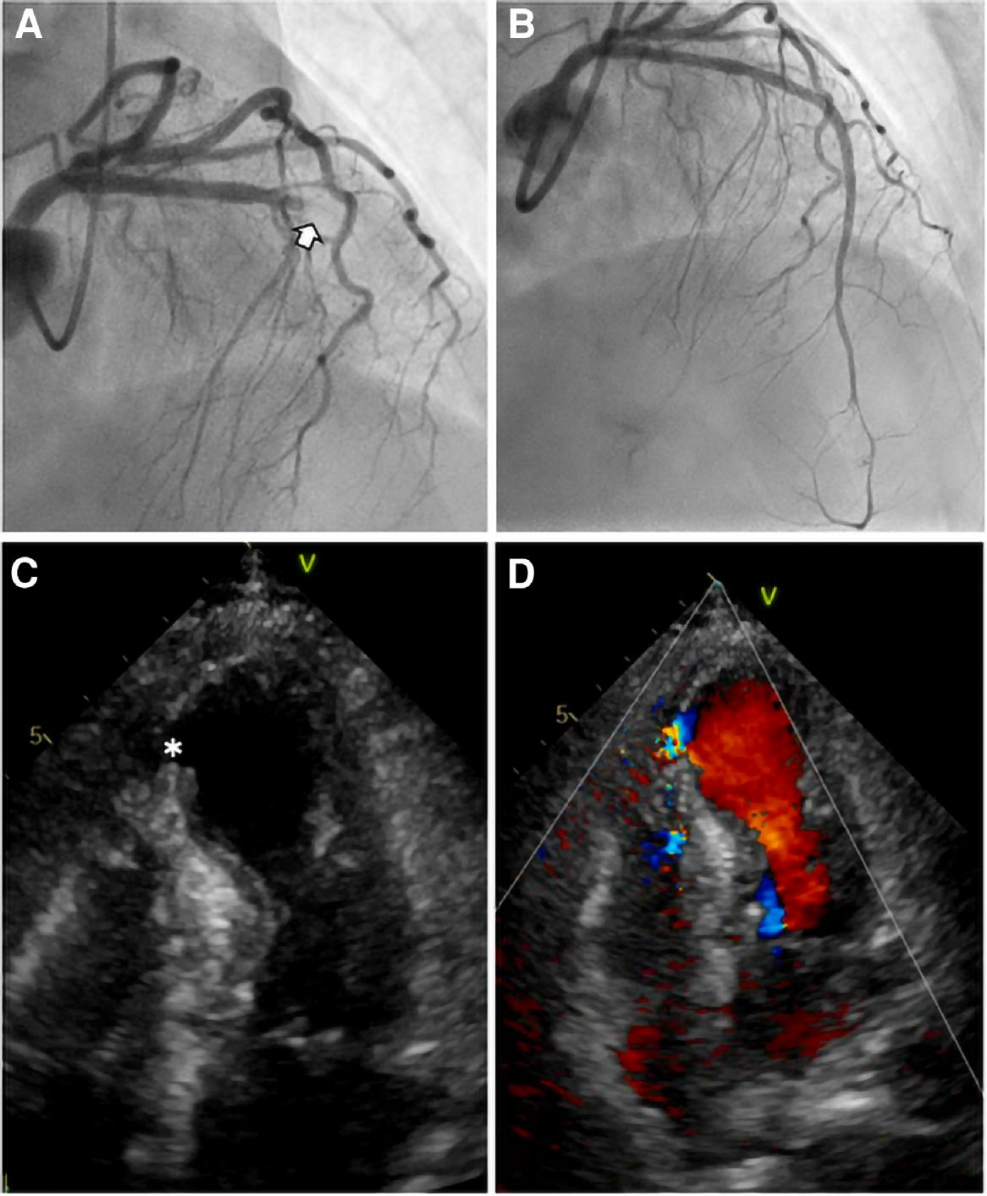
Imaging of acute left anterior descending artery infarction and ventricular septal rupture. (*A*) Coronary angiography showing total left anterior descending artery occlusion (arrow). (*B*) Successful revascularization following drug-eluting stent implantation. (*C*) Transthoracic echocardiography revealing an apical ventricular septal rupture (asterisk). (*D*) Colour Doppler image showing a left-to-right shunt through the ventricular septal rupture.
